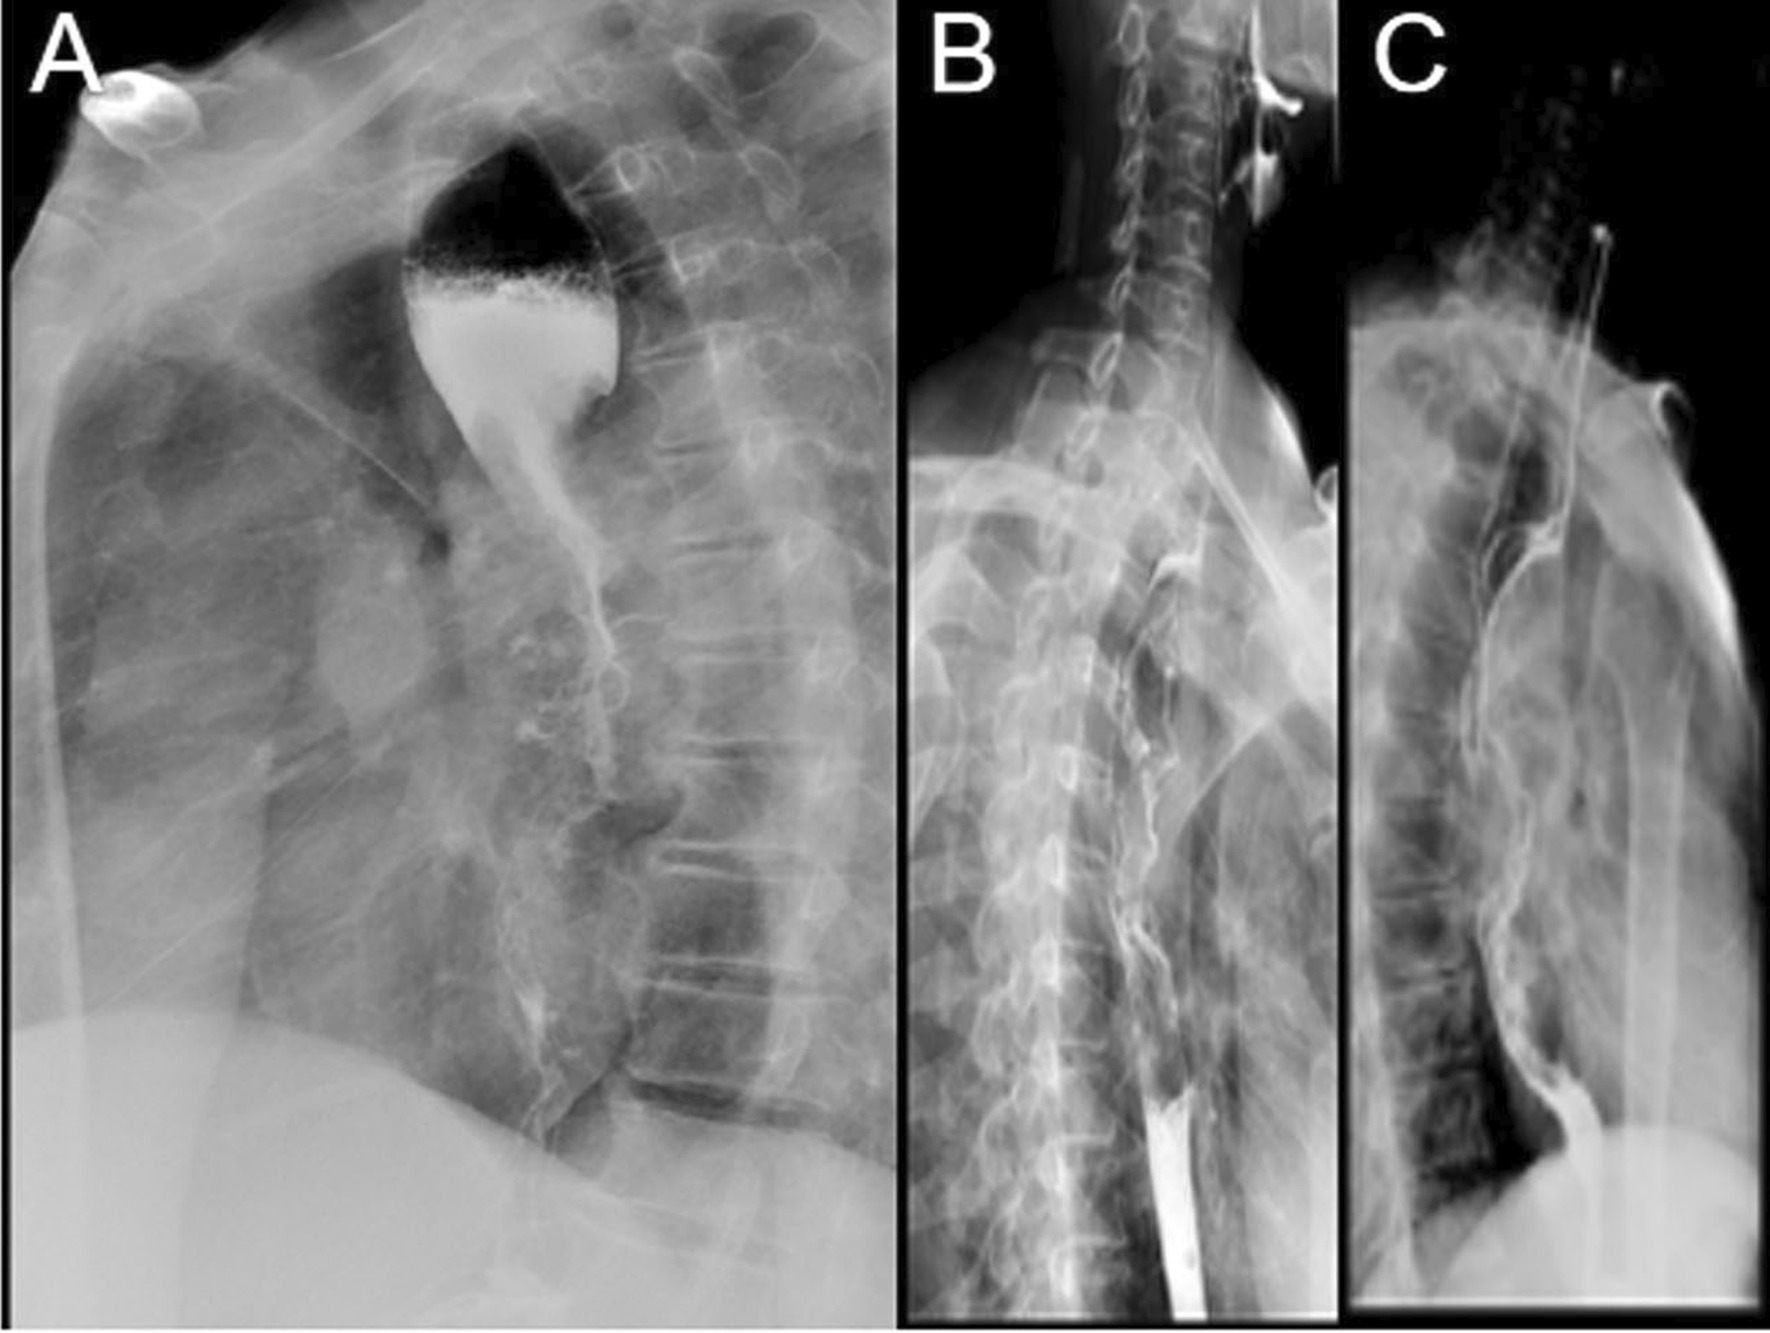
Preoperative upper gastrointestinal radiography manifestations of LCWEC. Preoperative upper gastrointestinal radiography displayed the filling defects caused by esophageal cancer in images A, B and C, which were 22.5 cm, 12.6 cm, and 18.3 cm, respectively, with almost complete obstruction of the esophagus
